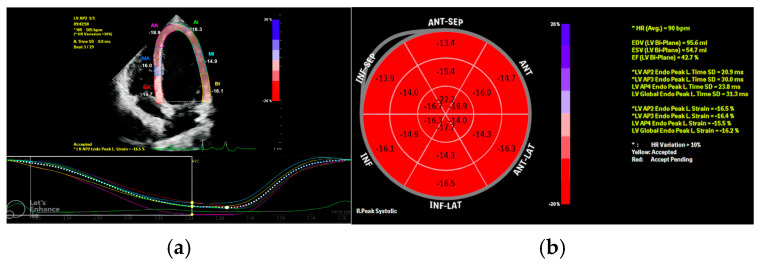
Representative two-dimensional speckle-tracking echocardiography images obtained from a 15-year-old female patient with a PVC burden of 6%. (a) shows the apical long-axis view with segmental longitudinal strain curves. (b) demonstrates the corresponding 17-segment bull’s-eye plot, with a global longitudinal strain (GLS) of −16.2%.
